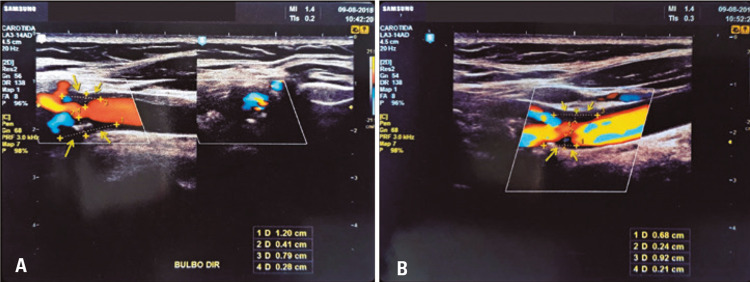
Doppler ultrasonography images (A and B) presenting atheromatous plaques (indicated by the arrows) in topography of carotid bifurcation on the right and left side, respectively
